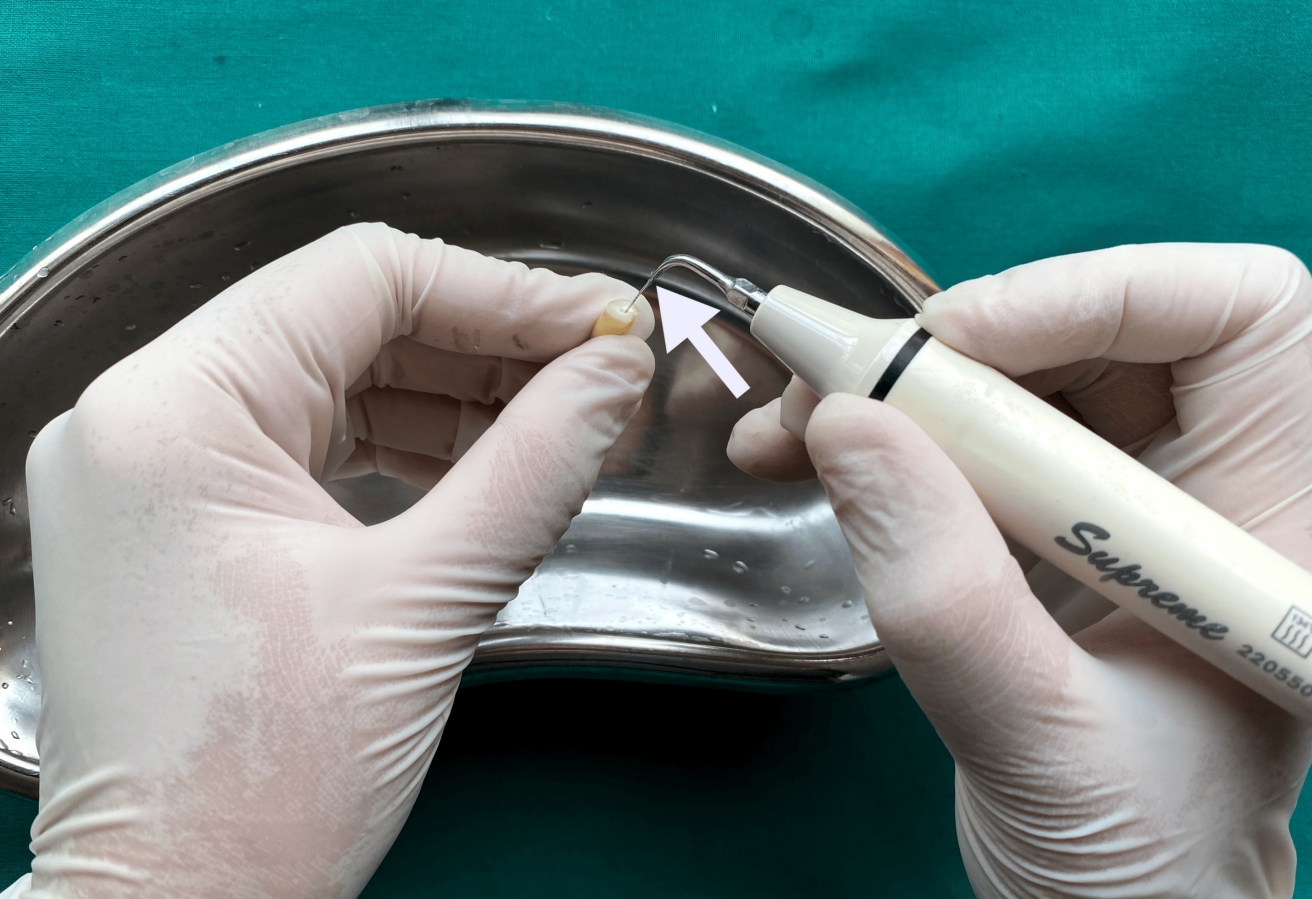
Irrigation activation using a passive ultrasonic irrigation tip
